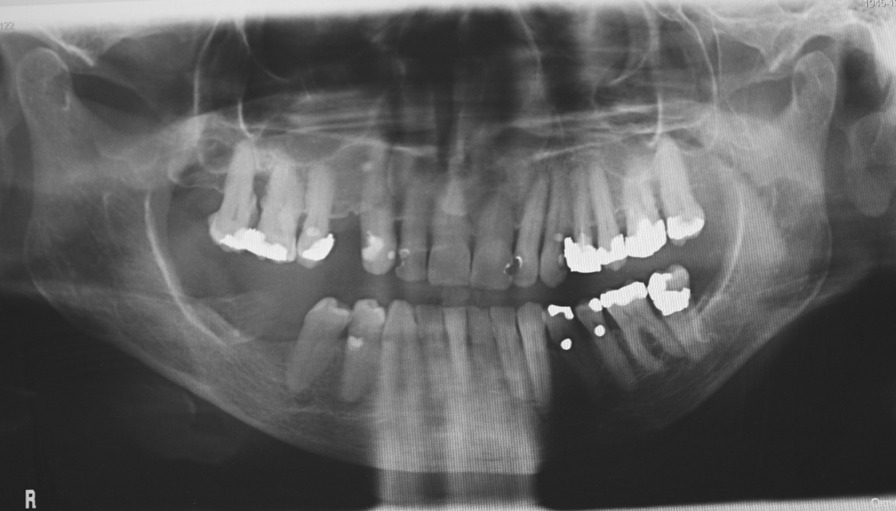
A panoramic radiograph taken in September 2017 with no signs of external cervical root resorption
